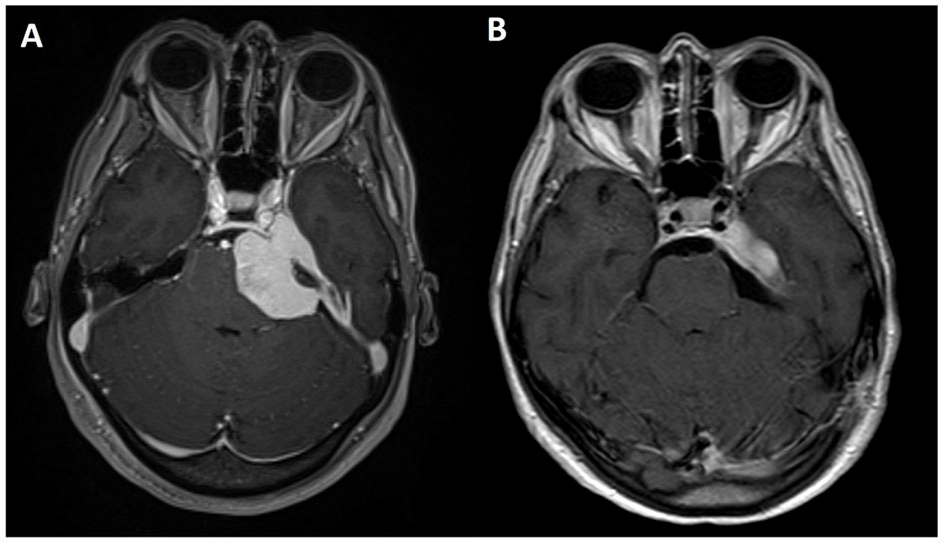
Axial view of T1-weighted MRI sequences with gadolinium: (A) preoperative and (B) postoperative.
